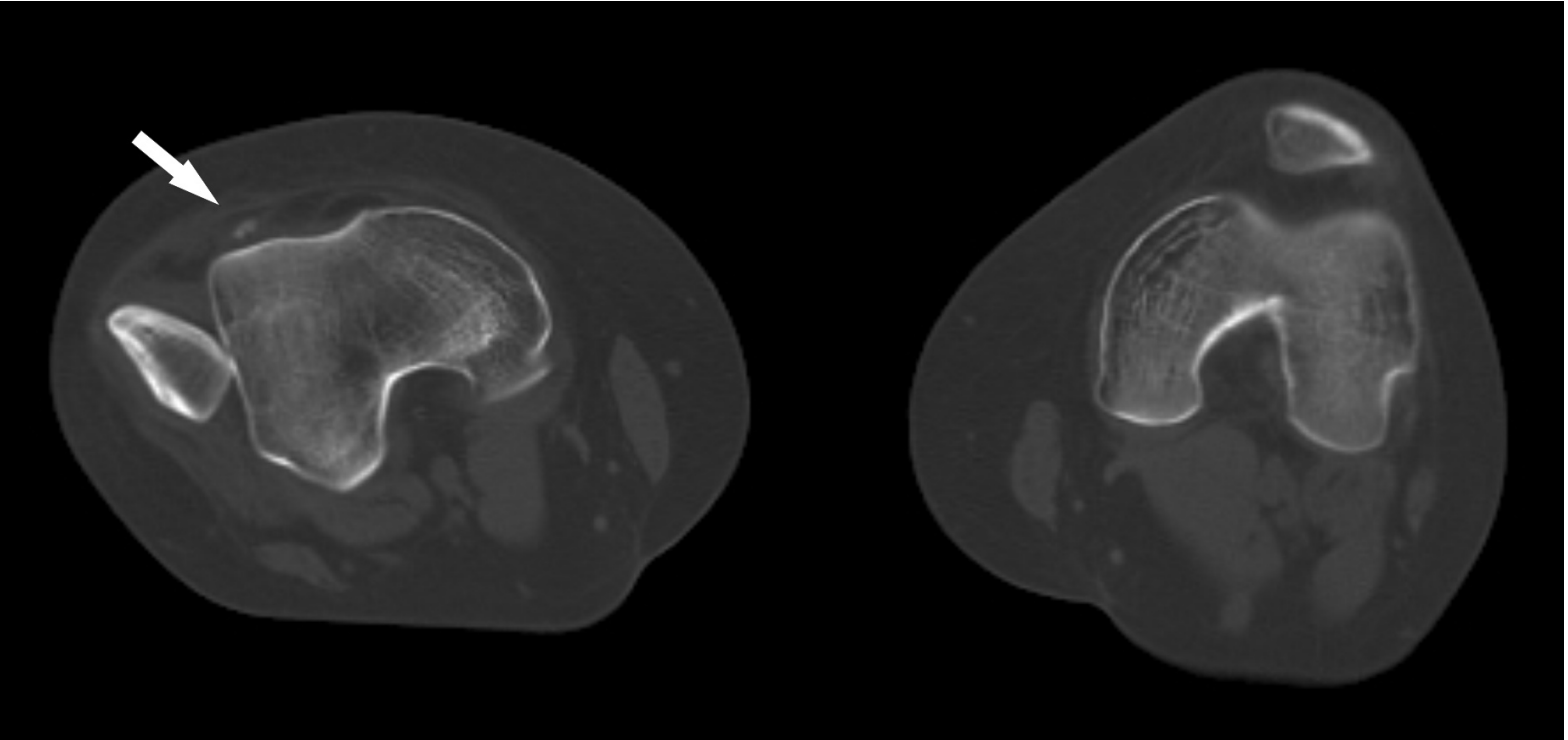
Computed tomography scan with axial image through both knees showing patellar dislocation on the right side. The arrow shows a loose body.
